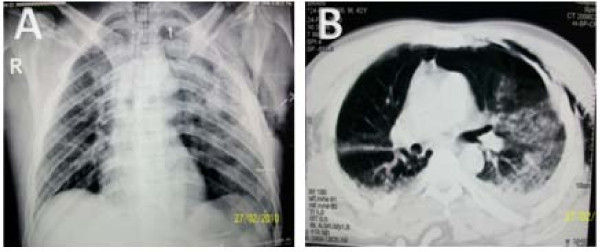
AP chest X-ray revealing evidence of bilateral lung contusions and left subcutaneous emphesema (Panel A). Chest CT confirmed both the lung contusions and the subcutaneous emphesema and demonstrated a left sided pneumothorax not initially appearing on the anteroposterior chest Xray (Panel B).
